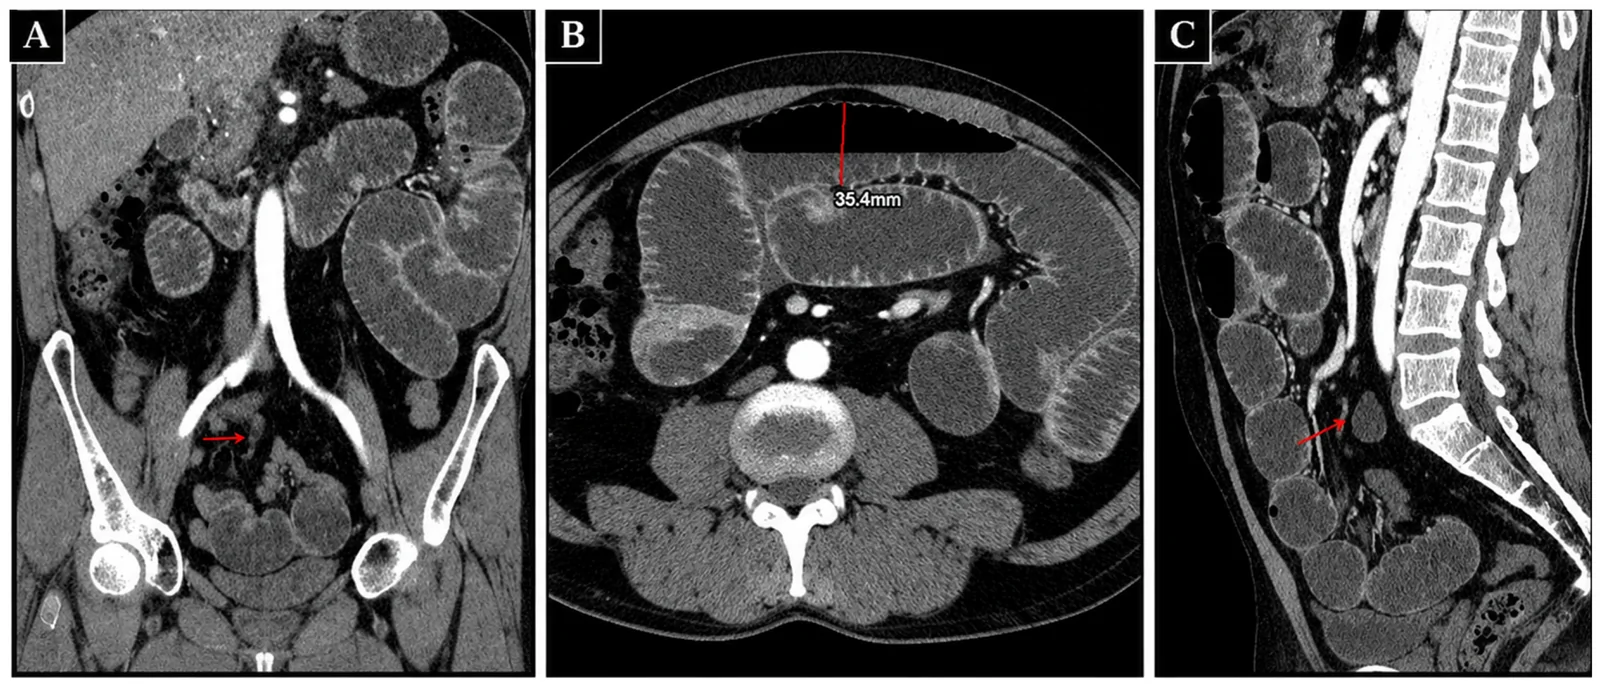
Contrast-enhanced computed tomography findings in small bowel obstruction. (A) Coronal contrast-enhanced CT demonstrating multiple dilated small bowel loops, with the arrow indicating the transition point in the right iliac fossa. (B) Axial contrast-enhanced CT showing a dilated small bowel loop measuring approximately 35.4 mm, with an air-fluid level. (C) Sagittal reformatted contrast-enhanced CT demonstrating the transition point (arrow), with dilated proximal and collapsed distal small bowel loops.
